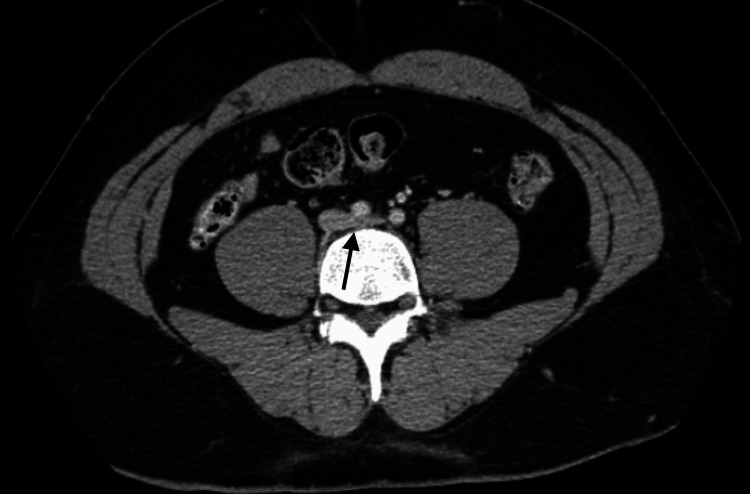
The CT angiogram of the abdomen/pelvis with and without contrast showing a slight impression upon the left iliac vein by the right iliac artery consistent with the clinical diagnosis of MTS CT: computed tomography; MTS: May-Thurner syndrome
